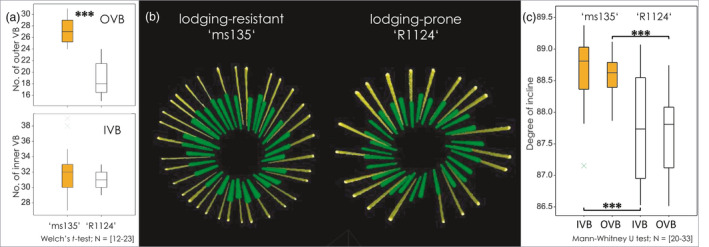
The arrangement of vascular bundles in lodging‐resistant (‘ms135’) and lodging‐prone line (‘R1124’). (a) Number of outer and inner vascular bundles (OVB; IVB). (b) Model of individual vascular bundles in inner (green) and outer (yellow) vascular ring by MRI‐based 3D modelling. (c) Degree of the incline of vascular bundles. Welch’s *t*‐test (a)/Mann–Whitney (c); P* < 0.05; *P* < 0.01; P* < 0.001.
